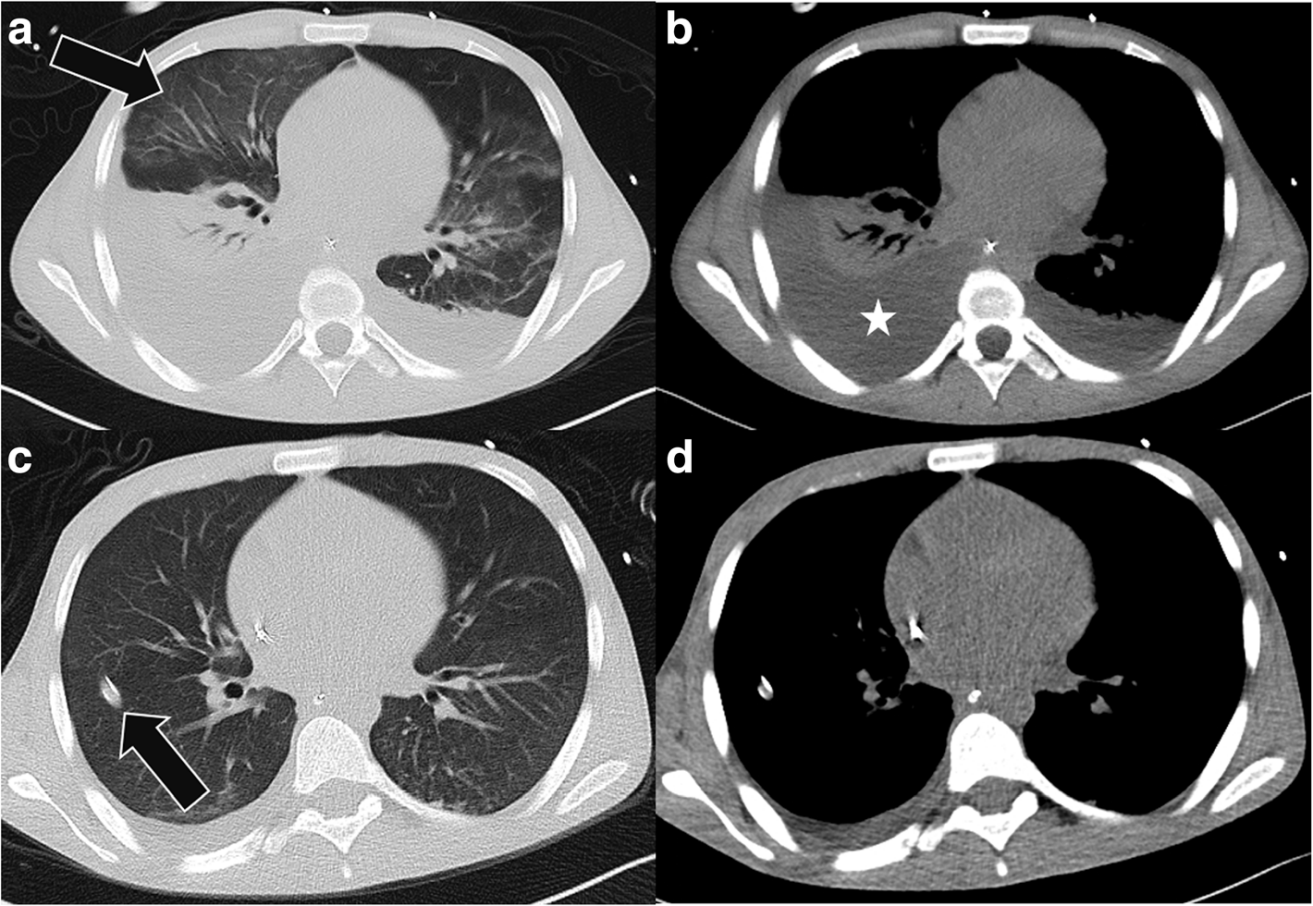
a-d Chest CT scans of the patient before (top row) and after (bottom row) therapy of pneumonia: top row shows ground glass opacities (a, arrow) as a sign for an atypical infection with large pleural effusions (b, *) and lung compression; lower row demonstrates resolving pulmonary infection (c) and only residual pleural effusion (d). A chest tube was inserted to reduce the effusion (c, arrow)
